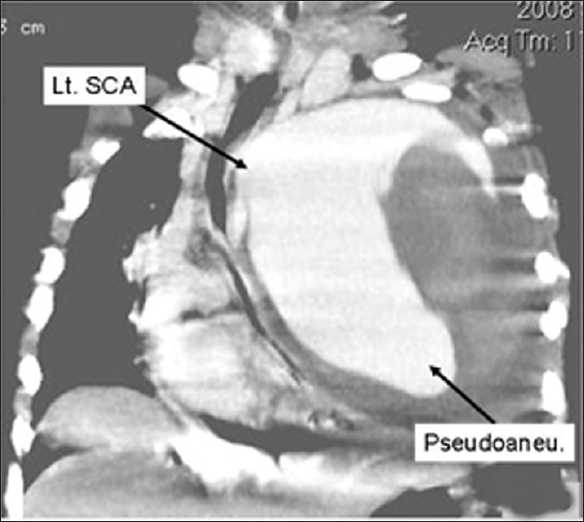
CT angiography (coronal reconstruction) showing large pseudoaneurysm arising from outer wall of left subclavian artery
